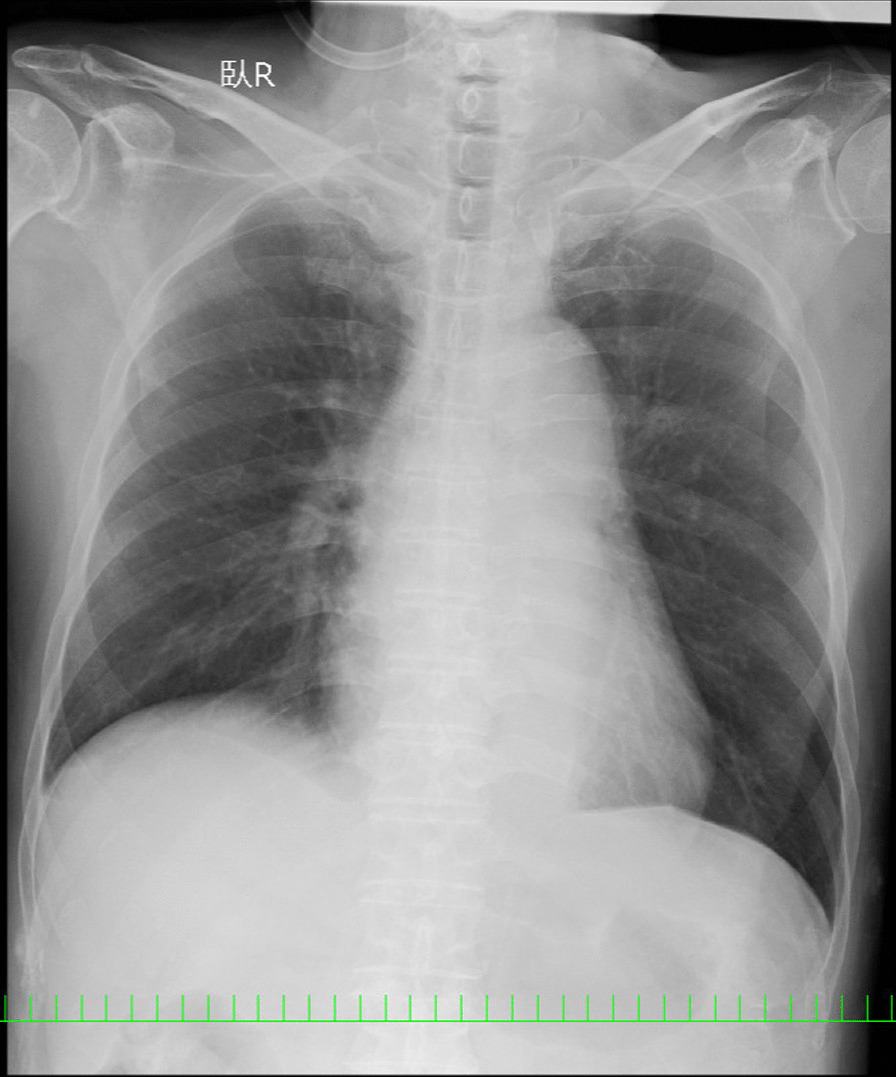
Postoperative chest X-ray
